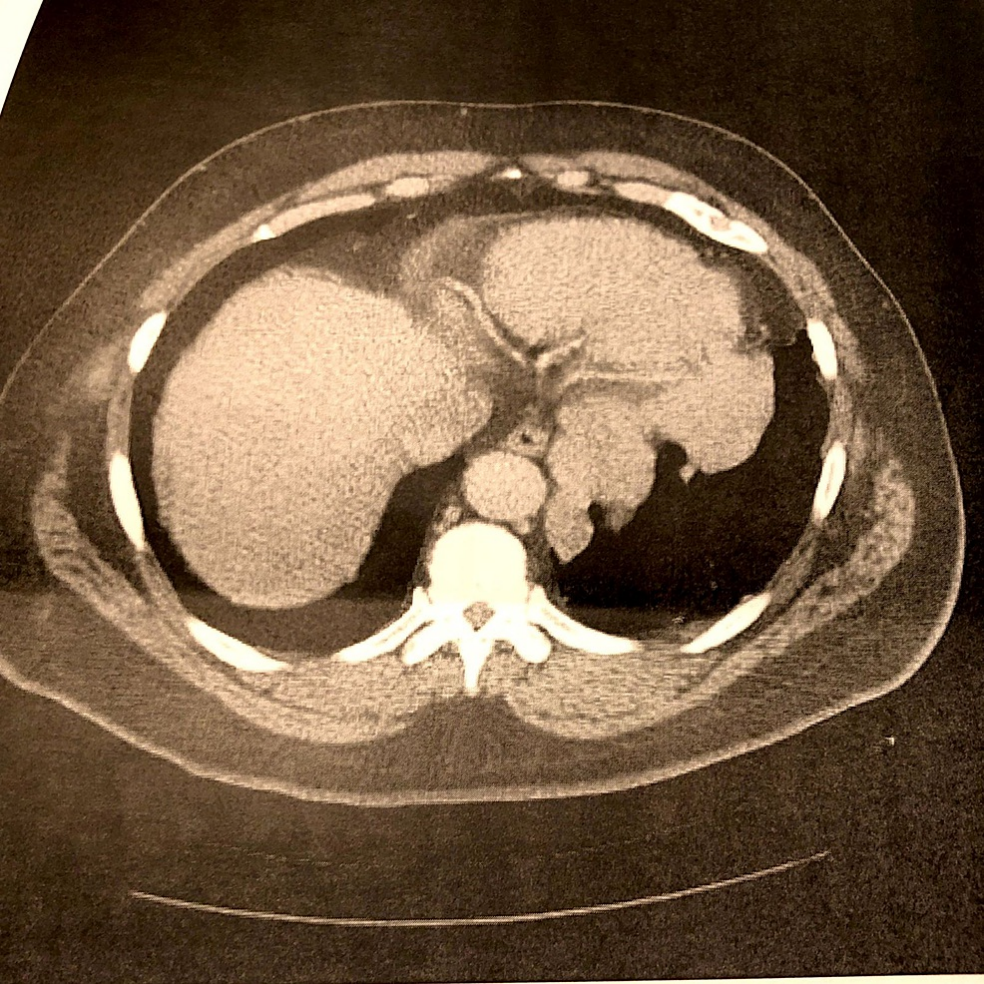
CT abdomen and pelvis w/contrast. Multiple left lower masses are seen.
